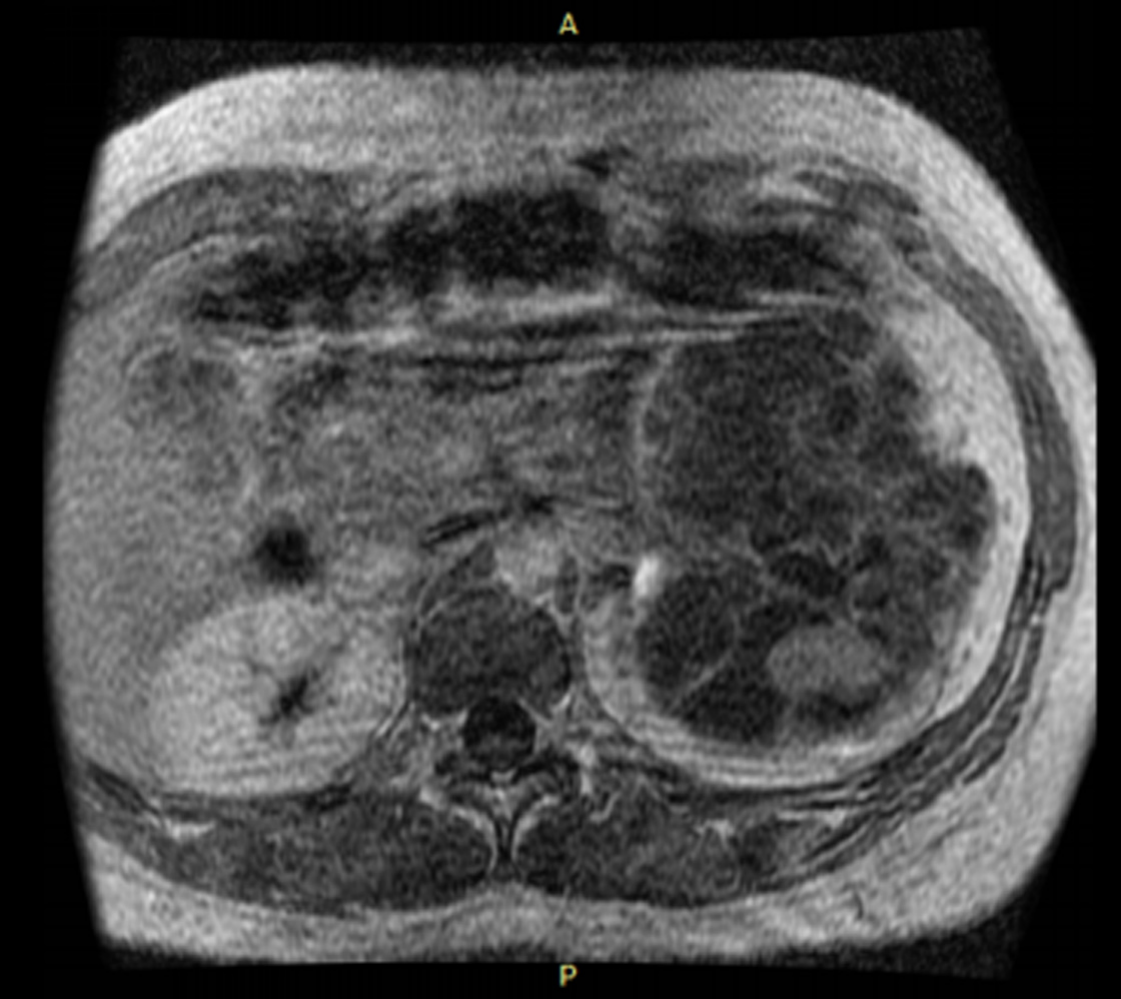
Magnetic resonance image of our first patient showing the complex cystic left renal mass.
